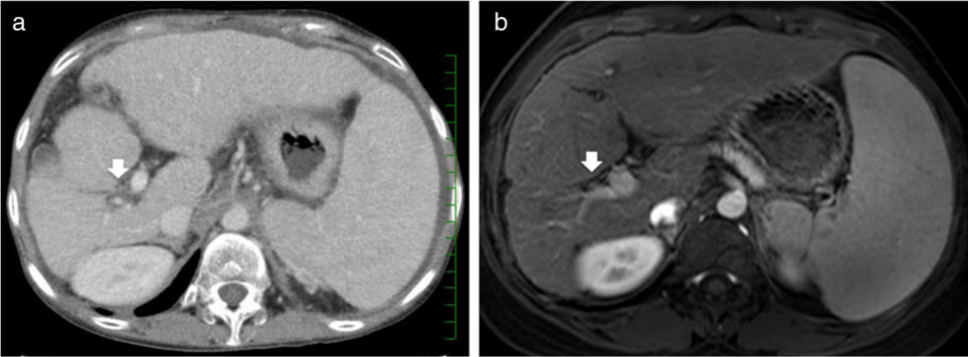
Chronological changes in radiologic findings of the liver. Enhanced abdominal computed tomography just before LDLT (a) and enhanced abdominal magnetic resonance imaging at 25 years of age (b). The progression of atrophy of the right lobe and severe stricture of the right portal vein (*white arrow*) is revealed
